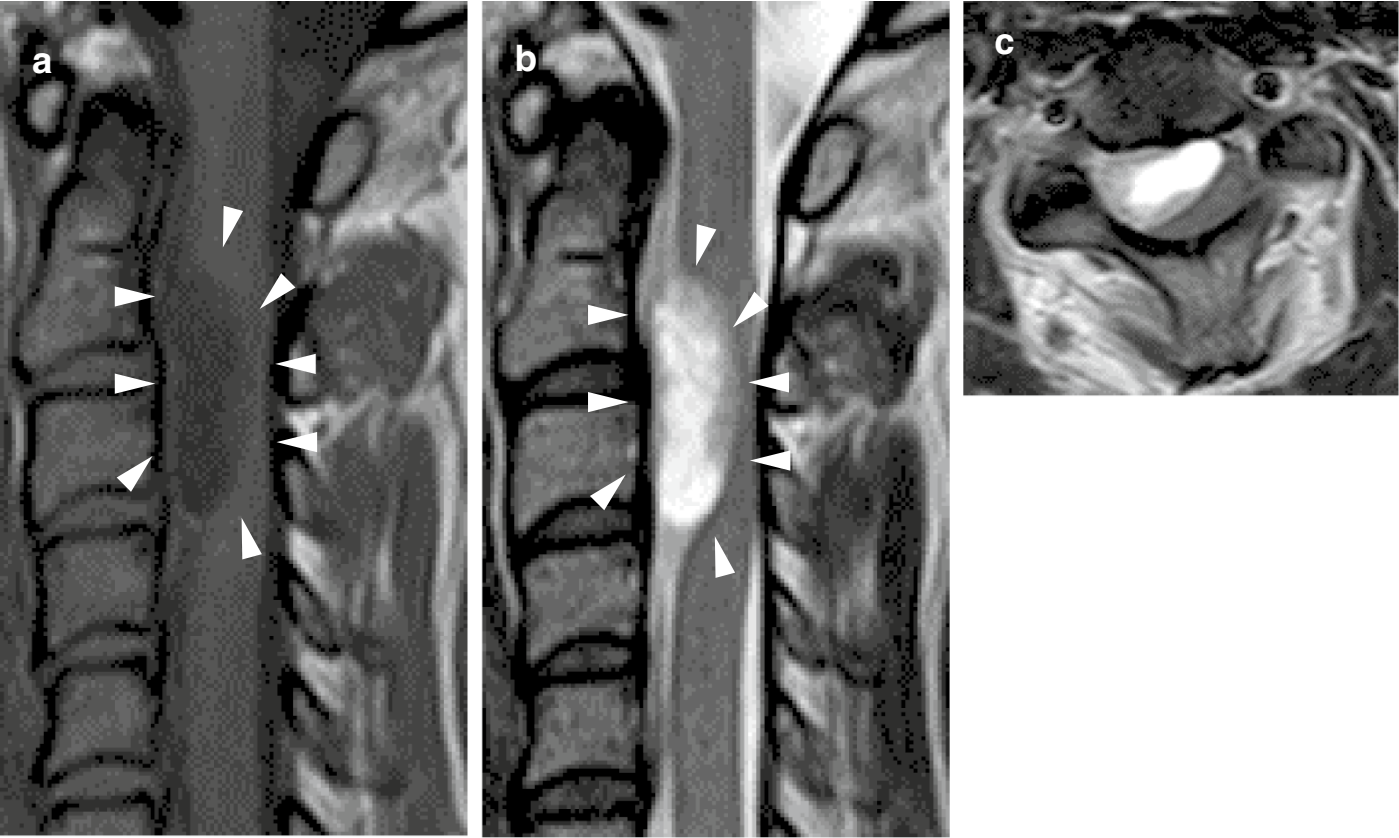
Preoperative magnetic resonance imaging (MRI) of the cervical spine. a mid-sagittal section of a T1-weighted image; b mid-sagittal section of a T2-weighted image; and c an axial T2-weighted image at the C3 vertebra. Arrowheads indicate the location of the spinal tumor
